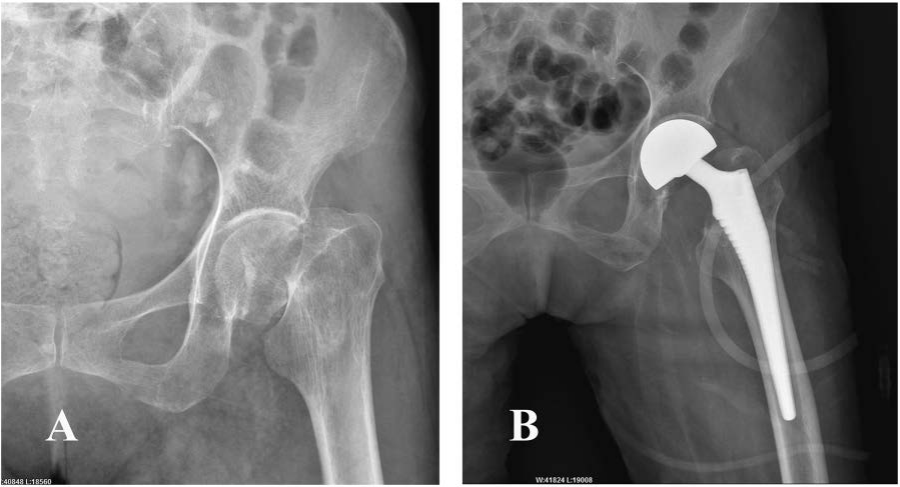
X.ray of a patient with a femoral neck fracture undergoing HA. Female, 76 years old, diagnosed with femoral neck fracture with sarcopenia (A) Preoperative X-ray (B) Postoperative X-ray.
